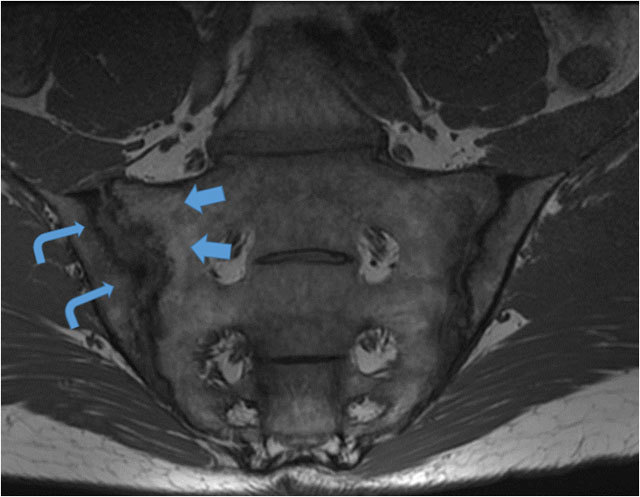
T1-W mid coronal oblique image through the SIJ in a 42-year-old male who presented with long standing low back pain, raised inflammatory markers and a positive HLAB27. Juxta-articular geographical fat infiltration (blue arrows) is noted as structural lesions, along the sacral margin of the SIJ. Pseudowidening of the joint on MRI with large elongated erosions along the iliac aspect and subarticular sclerosis (curved arrowheads) of the right SIJ.
